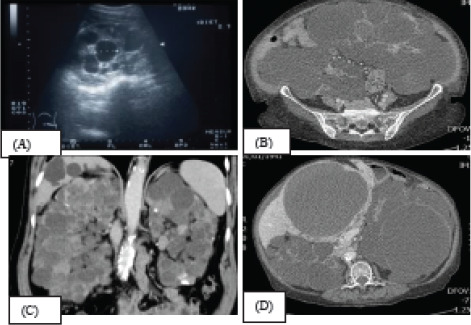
A) Ultrasound, CT, B) MR C) scan showing multiple renal cysts, and D) CT scan showing liver cysts.
